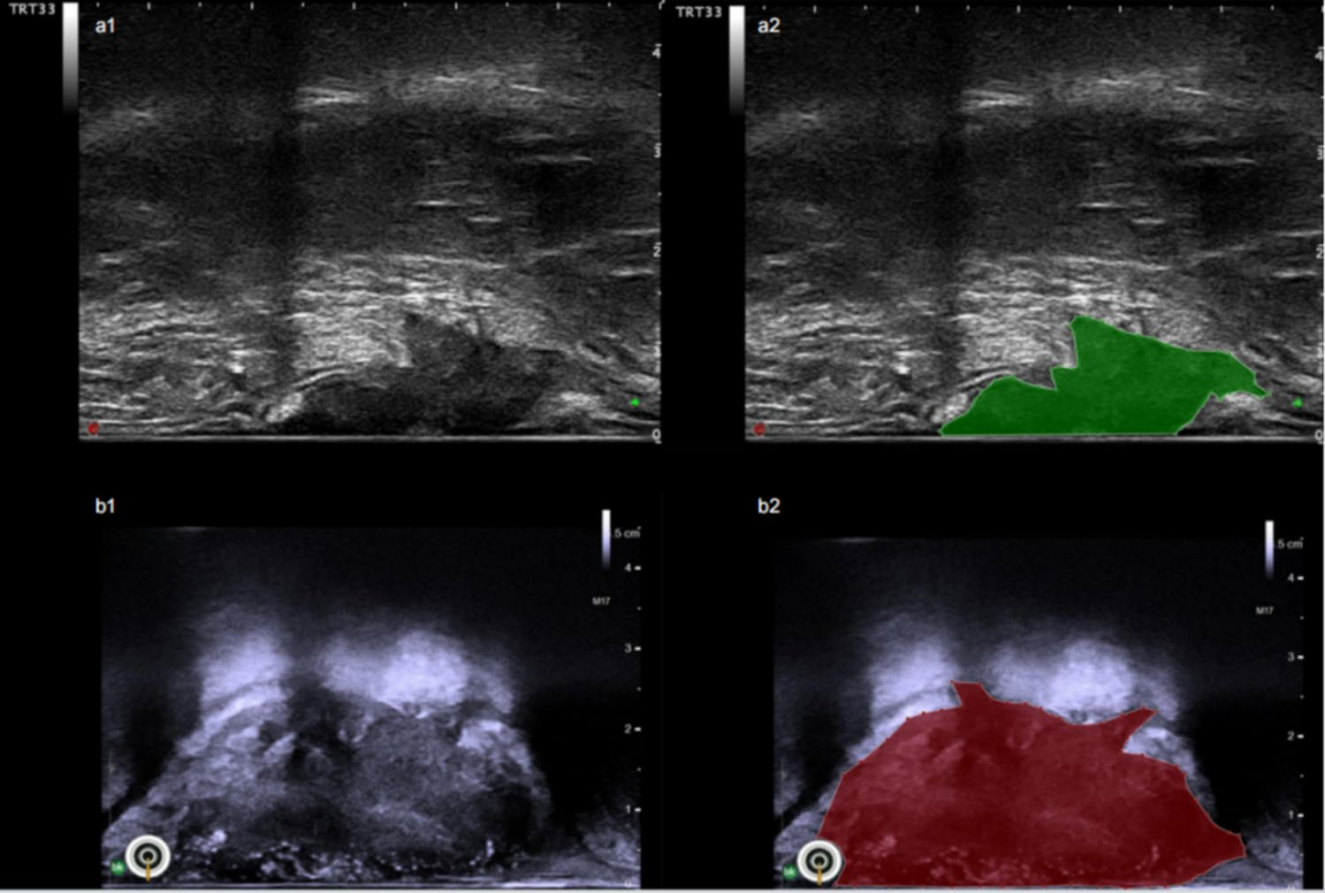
Delineation of the LARC lesions in the TRUS images. (a1-a2) Acquired by Biosound MyLab Twice ultrasound diagnostic system before NCRT, the TRUS image (a1) and mask image (a2) of the lesion (TRG 1). (b1-b2) Acquired by BK Medical BK5000 ultrasound diagnostic system after NCRT, the TRUS image (b1) and mask image (b2) of the lesion (TRG 3)
